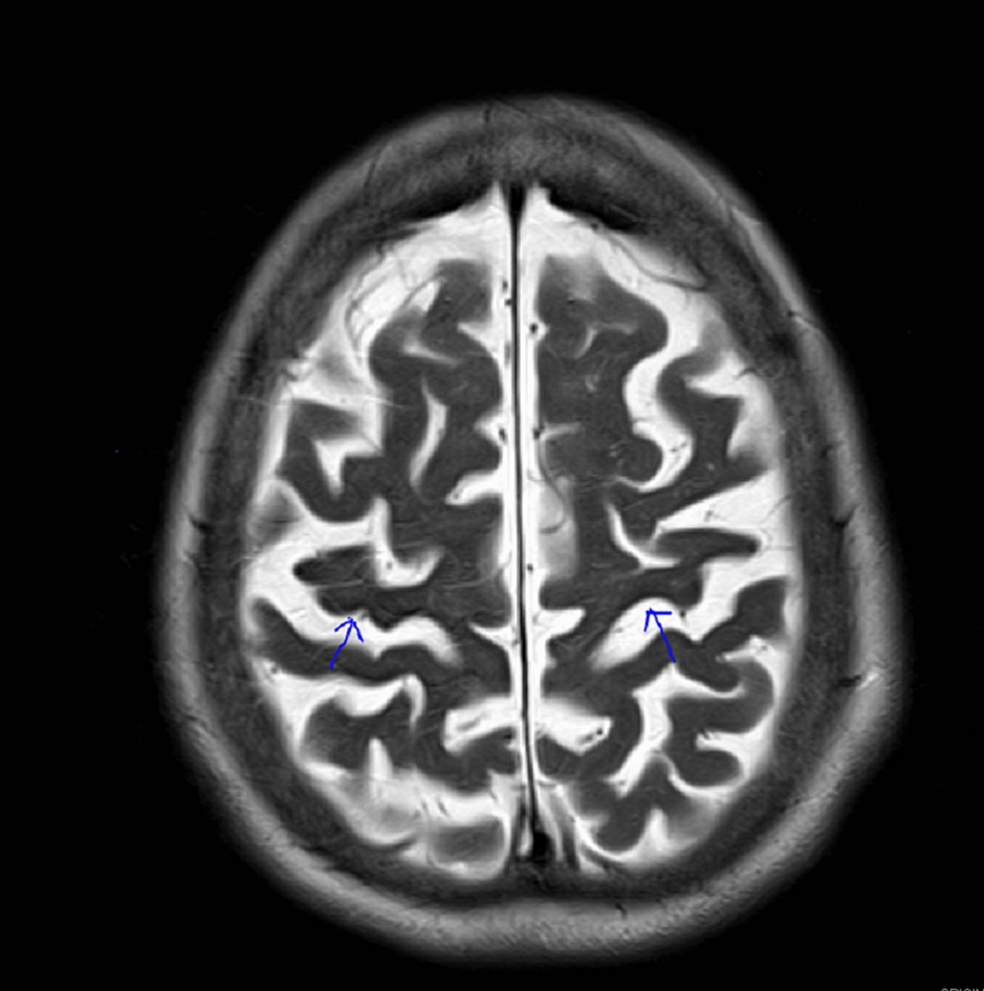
Bilateral hypointense signals on T2 sequence of brain MRI in the pre-central region (blue arrows) T2: transverse relaxation time, MRI: magnetic resonance imaging
